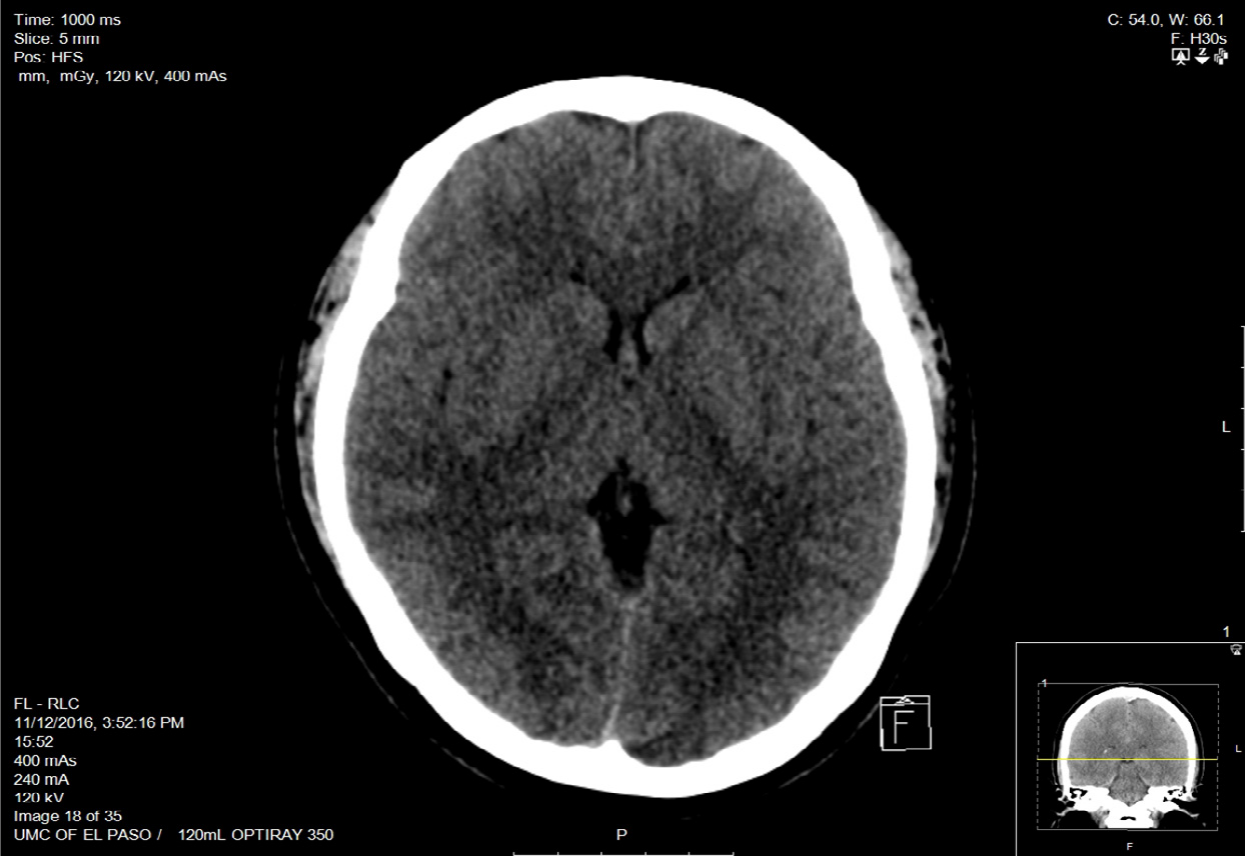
Computed tomography (CT) head and brain without contrast is negative for acute intracranial pathology.
